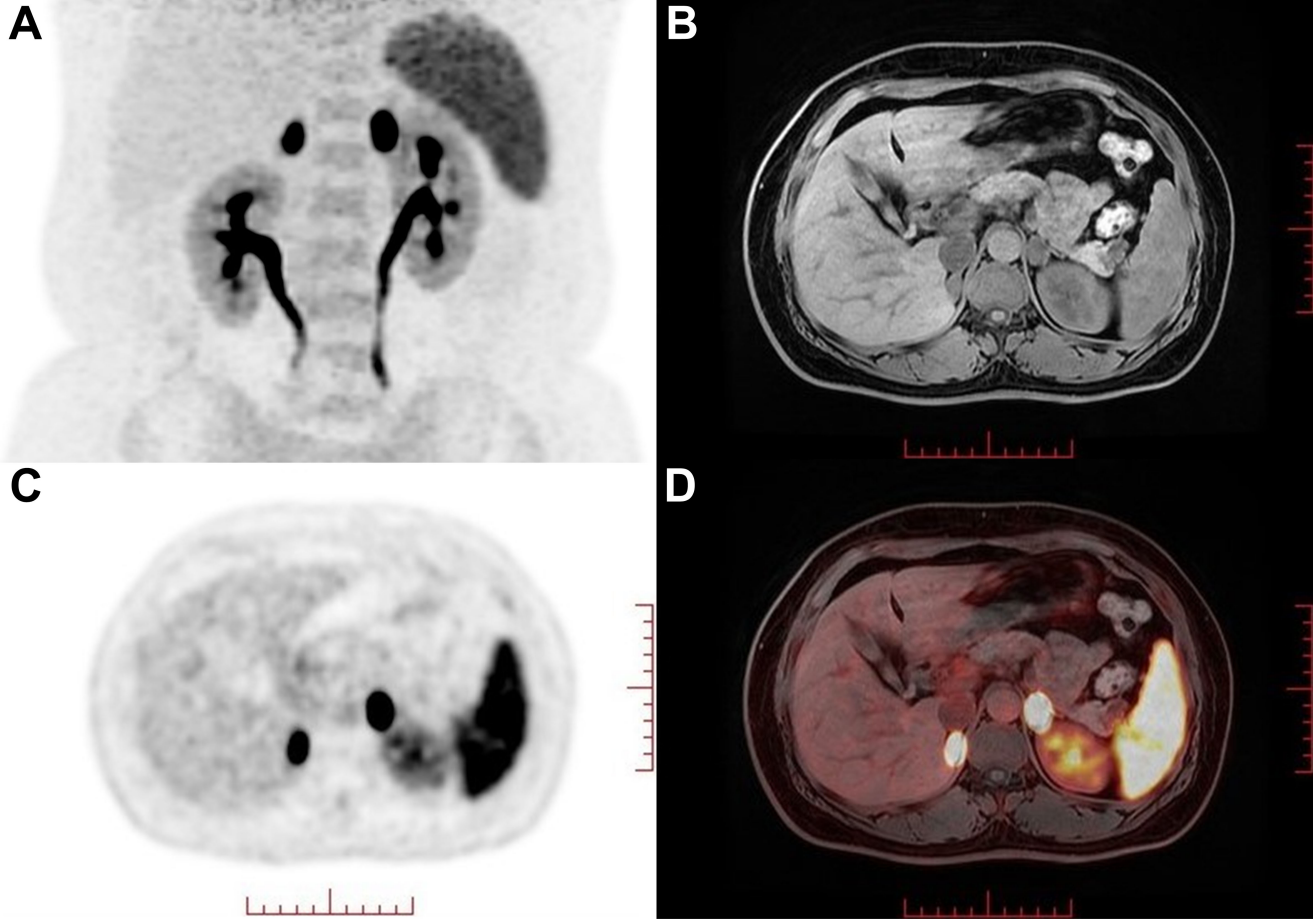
^68^Ga-Pentixafor PET/MR nuclear imaging. (A) MIP map showed foci in bilateral adrenal areas. (B-D) MRI and fusion images showed a 19×15 mm (right) and 18×16 mm(left) nodule with intense uptake (SUVmax values of 29.1 and 46.0 on the right and left sides, respectively) in each of the adrenal medial limbs of the bilateral adrenal glands.
